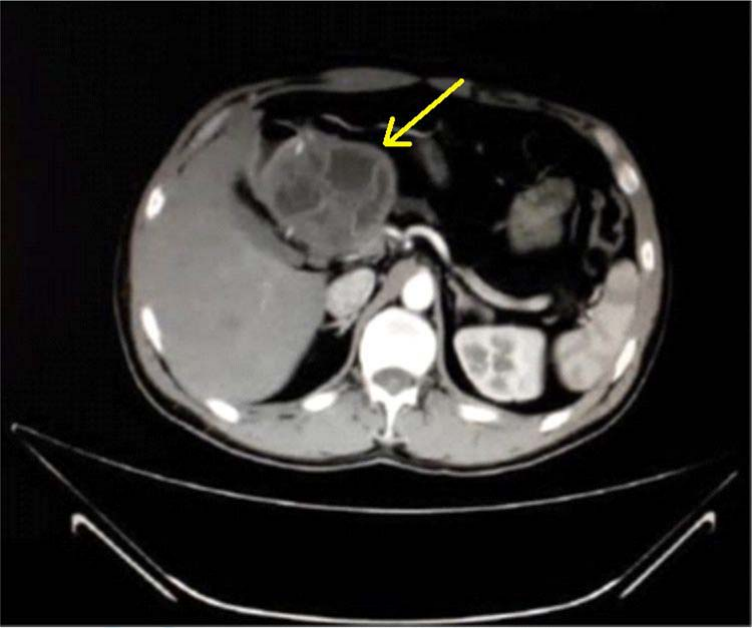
Axial contrast-enhanced CT scan of the abdomen demonstrating a well-defined solitary cystic lesion in the head of the pancreas with a diameter of (9 cm).
